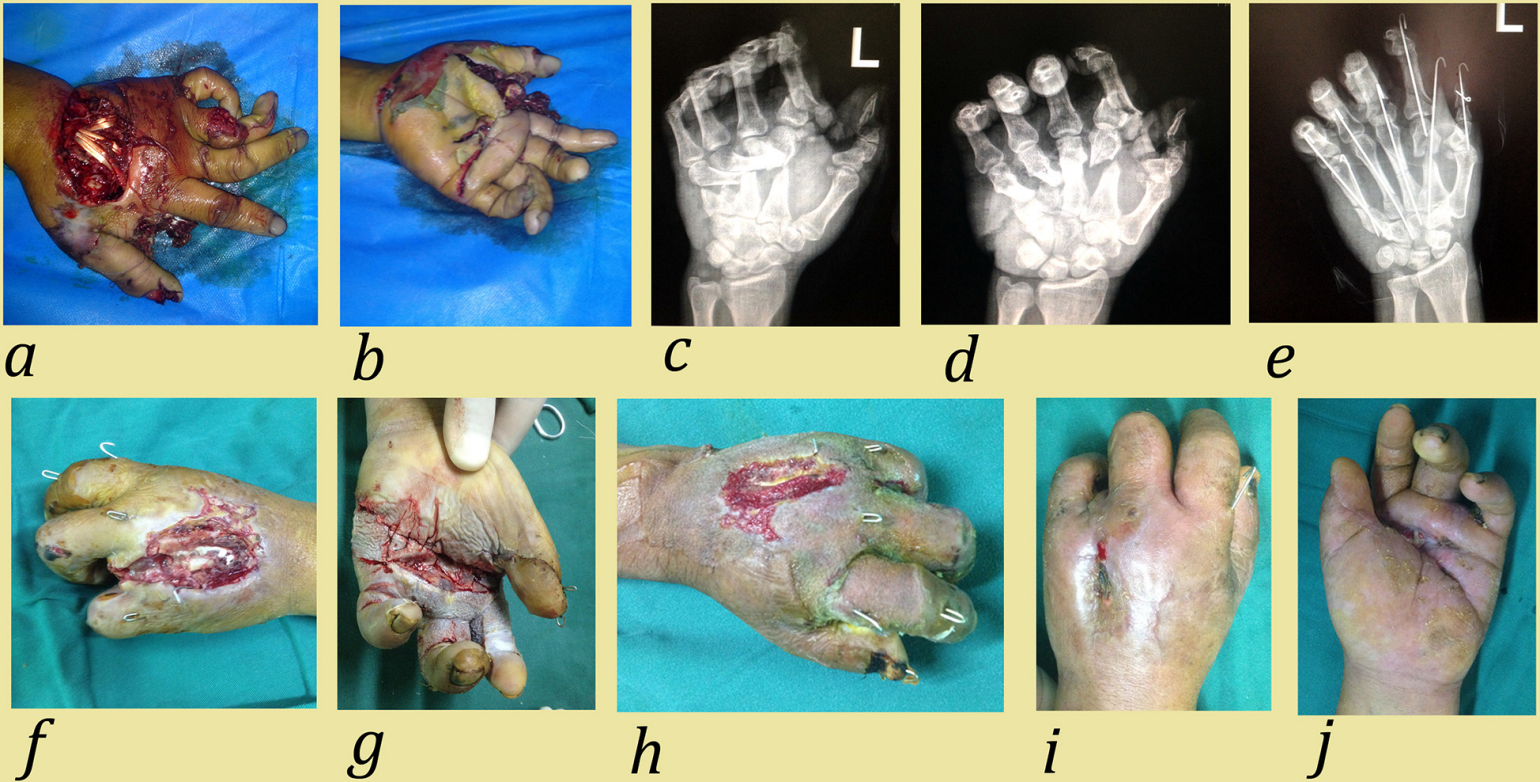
Case 1: A 51-year-old man suffered a corn-husker machine injury to his left hand involving metacarpal fracture, phalangeal fracture, and neurovascular injury. (a,b) Preoperative view; (c,d) Preoperative anteroposterior and lateral radiographs; (e) Postoperative anteroposterior radiograph. Emergency surgical methods included: debridement and suturing, fracture reduction, K-wire fixation, and neurovascular and tendon repair; (f) Appearance 3 weeks after the first operation. The necrotic ring finger was removed and NPWT was applied to the wound; (g,h) Appearance 4 weeks after the first operation. After NPWT, granulation tissue grew and skin grafting was performed; (i,j) Final appearance of left hand. Due to wound infection, complete healing occurred eight weeks after the first operation.
